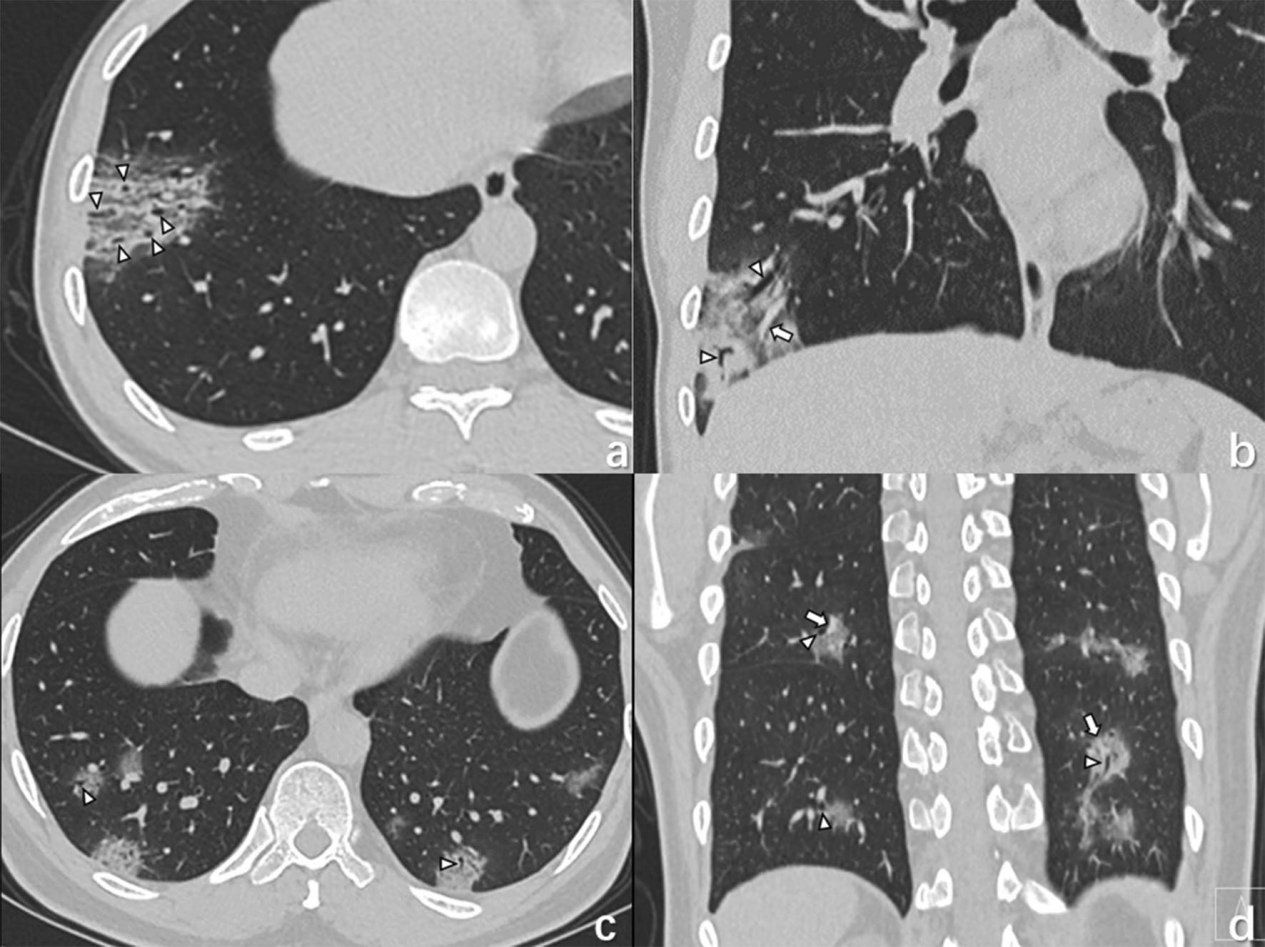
(a, b) A 35-year-old male with COVID-19 infection presenting fever and headache for 1 day. CT images shows small vascular enlargement (arrows) and bronchiolectasis (triangles) in GGO, as well as multiple GGOs in the subpleural areas of bilateral lung. (c, d) An 80-year old man with COVID-19 infection, who lives in Wuhan presented with fever and dry cough. CT images shows multiple patchy areas of GGOs as crazy-paving pattern with bronchiolectasis (triangles) and vascular enlargement (arrows).
